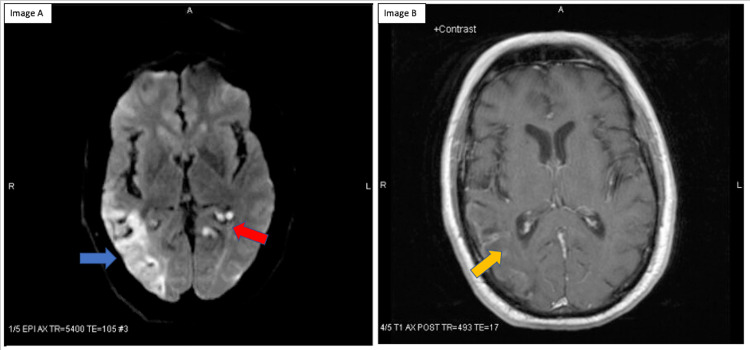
MRI brain of the patient. Image A: Diffusion-weighted imaging demonstrating multiple strokes in the left medial thalamus (red arrow), subacute right temporal-occipital infarct (blue arrow); Image B: T1 post TR displaying luxury perfusion of the subacute infarct.
